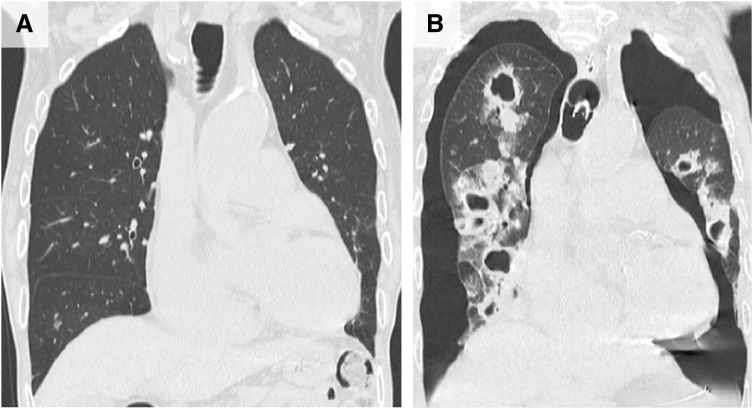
Coronal reconstructed chest CT images with lung window settings. (A) Preoperative CT showed no significant abnormalities in the lungs. (B) On POD 10, bilateral pneumothorax and multiple cavitary lesions were observed in both lung fields.
